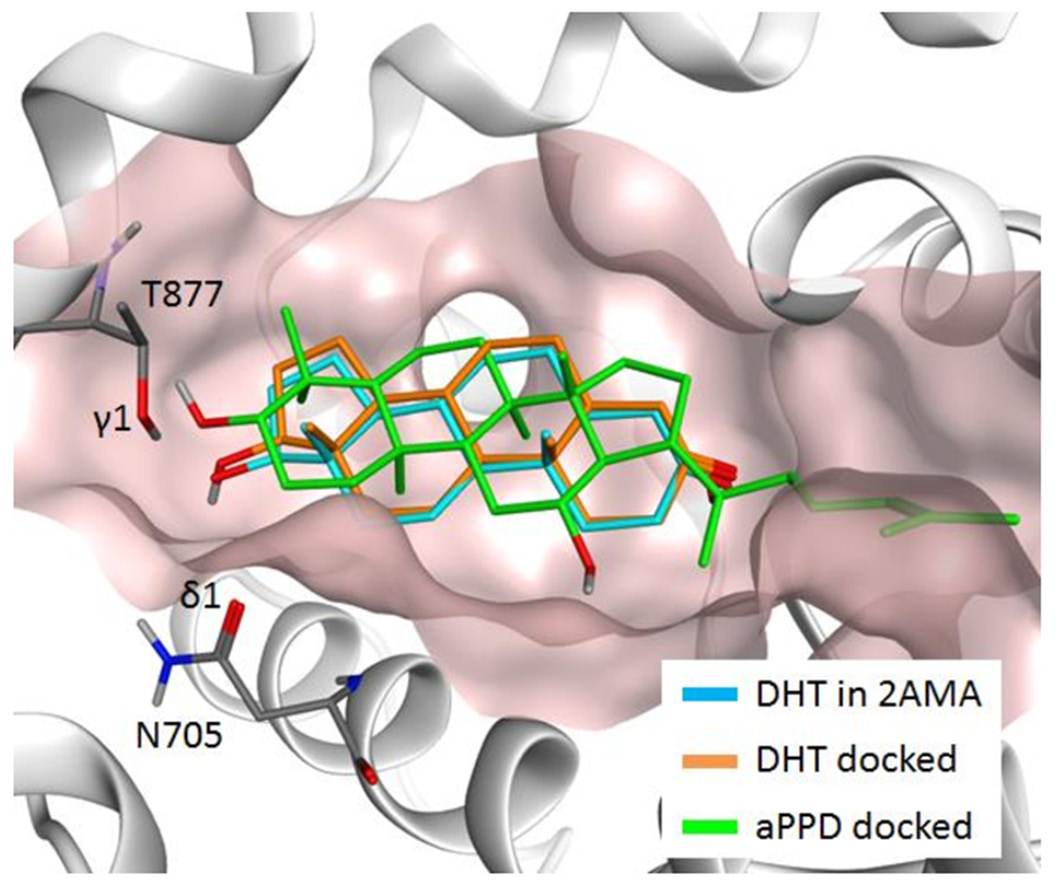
Predicted docking poses of dihydrotestosterone (DHT) (orange) and aPPD (green) in AR ABS, along with the DHT (light blue) in the 2AMA X-ray structure
